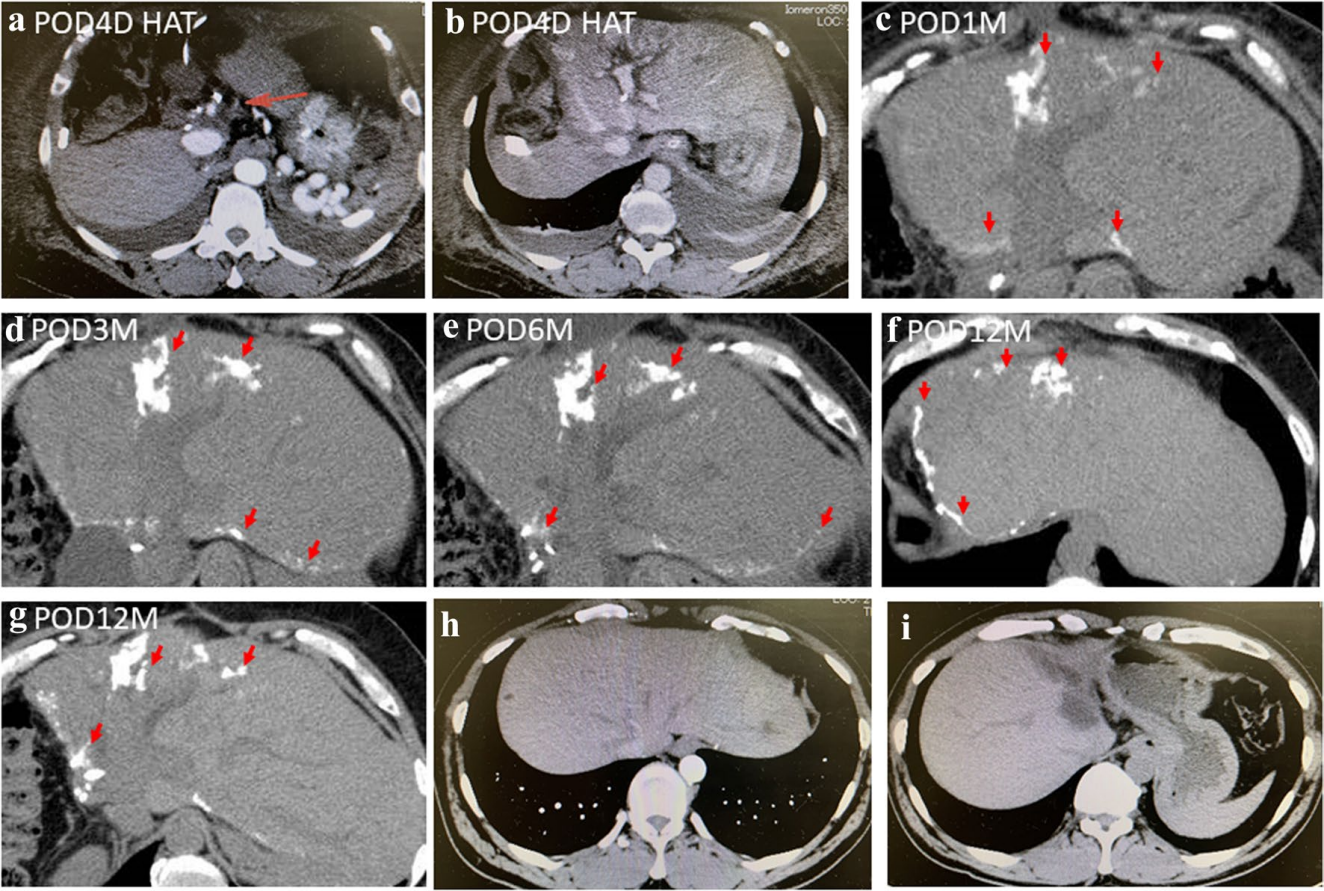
CT image from case 2 showing the accumulation of calcium in the wide range of the liver. a, b CT images showed the hepatic artery thrombosis on POD4. c One month after LDLT, extensive calcification of the liver graft was found during the reexamination. d Three months and e 6 months after LDLT, the area of calcification in the liver had increased compared to before. f, g CT images showed extensive graft calcification 1 year after LDLT. h, i Preoperative and postoperative images showed no presence of liver calcification in the donor liver
